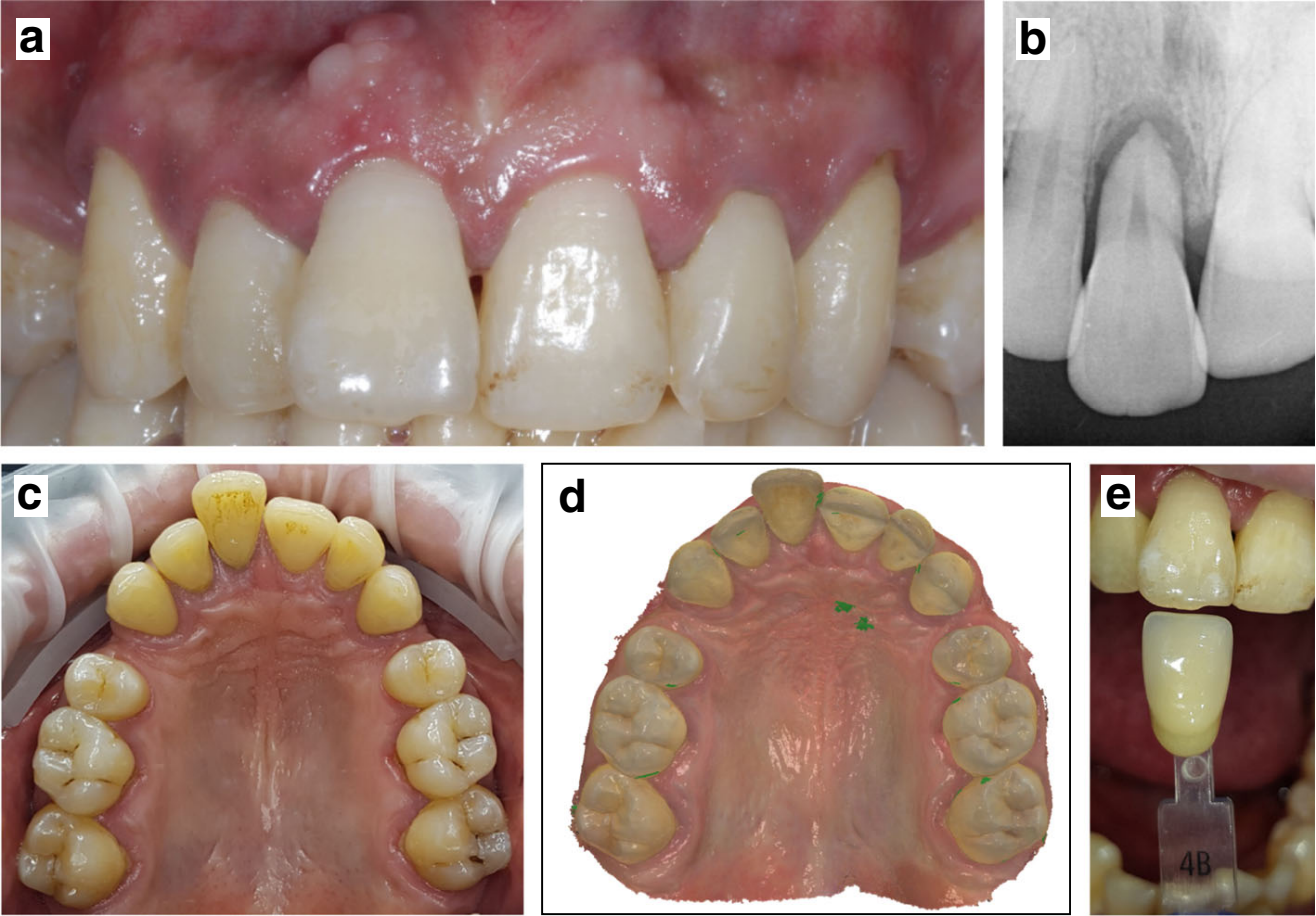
Initial situation. The patient consulted for a pain caused by the mobility of the maxillary right central incisor (a). An intraoral radiography confirmed the partial extrusion of the tooth (b). A digital impression of the patient’s maxillary arches was made with an intraoral scanner (c and d) and color registration was performed (e)
